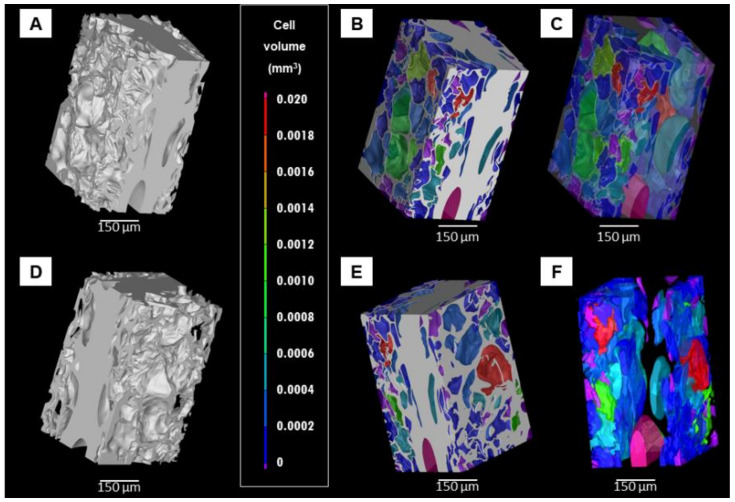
Three-dimensional (3D) virtual reconstructions of a lyophilized PVP hydrogel obtained by nano-CT. (A,D) Two different views of the material. (B,C,E,F) Pore volume distribution in colour-scale, images C and F are the same than images B and E, respectively, but the material was defined transparent for better observation of the pore morphology and distribution.
